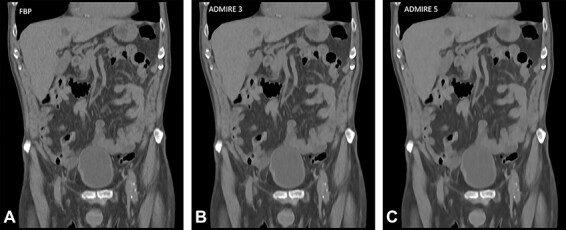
Examples of image quality comparisons in full-dose abdominal CT (quality reference (Qref) 140 mAs) from a study patient^(10)^, reconstructed with FBP and ADMIRE (Siemens Healthineers) at Strengths 3 and 5, out of 5 available strengths.
